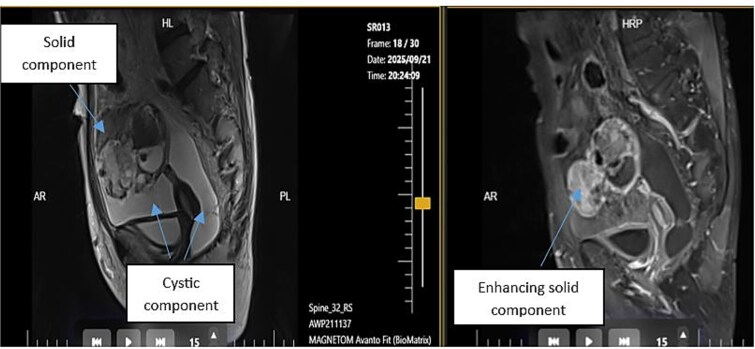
Pelvic MRI: (left) sagittal T2-weighted image showing a left adnexal complex cystic-solid mass; (right) fat-suppressed contrast-enhanced T1-weighted image showing enhancing solid component (arrow)—O-RADS 5.
